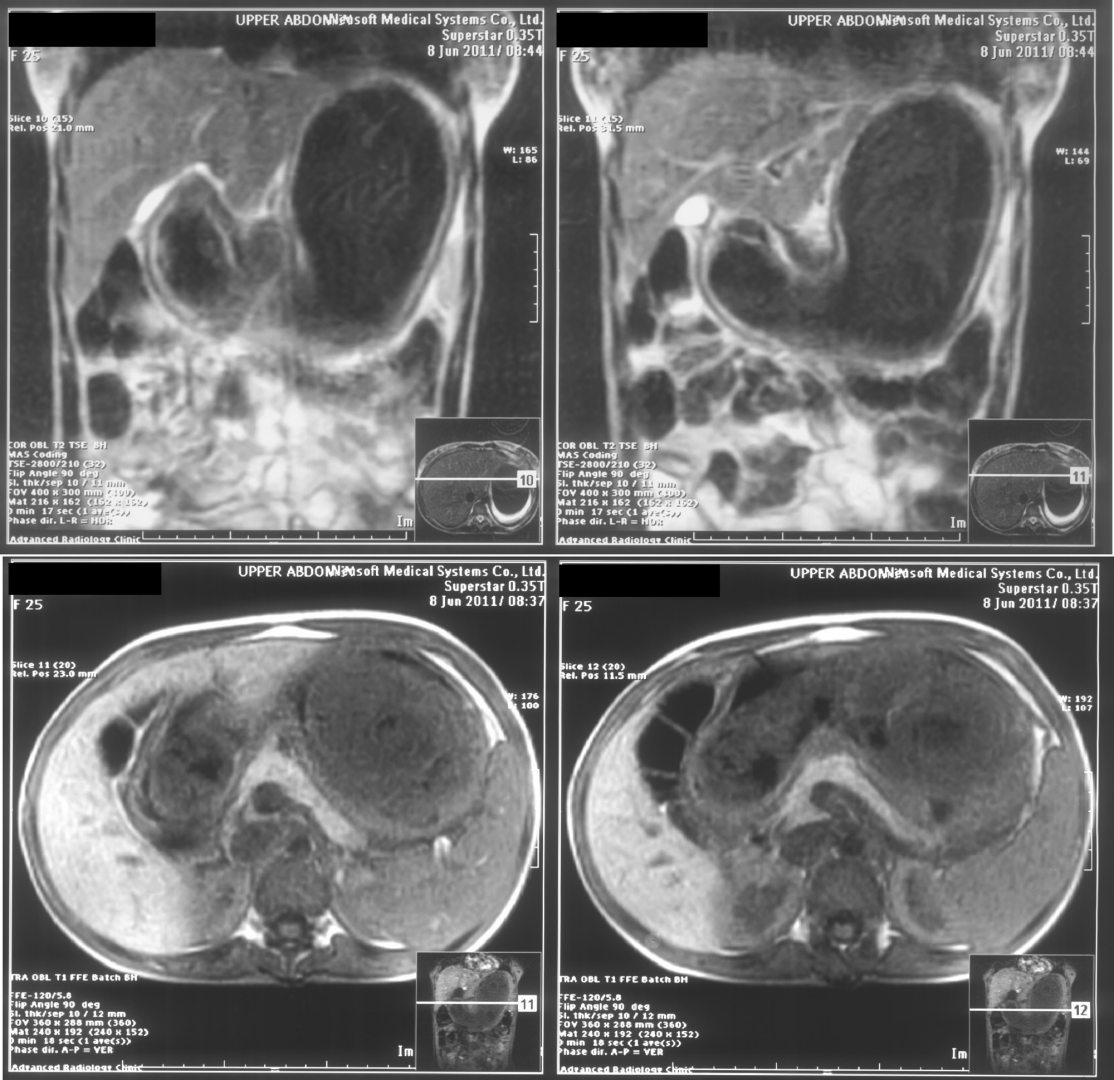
Magnetic resonance imaging (MRI) views showing trichobezoar.
